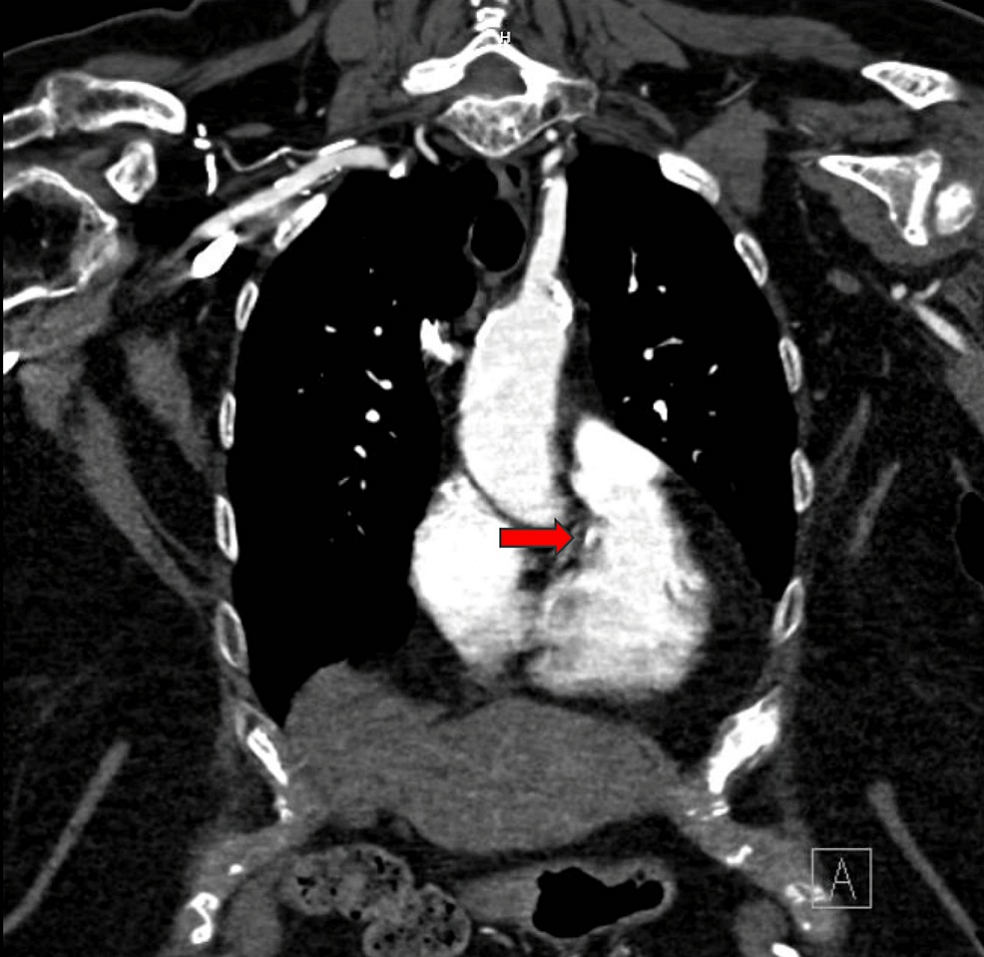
Computed tomography (CT) of the chest with contrast in the coronal plane showing a focal calcified lesion adjacent to the ostia of the right coronary artery at the root of the ascending aorta
